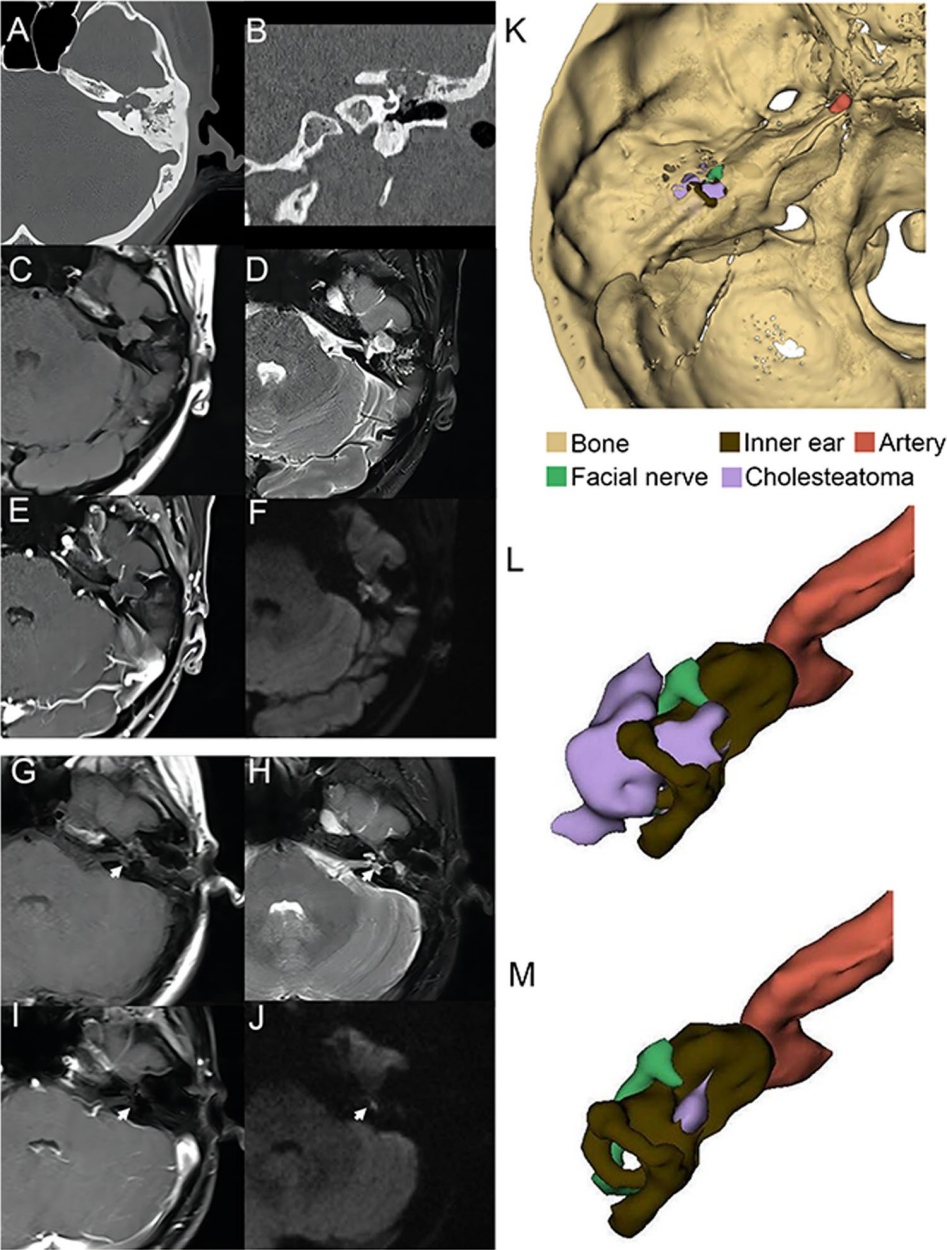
A case of cholesteatoma recurrence on imaging. A, B Left petrous bone lesion on preoperative CT involving the epitympanum, middle skull base, labyrinth, facial nerve, and internal auditory canal. C The mass had iso-intensity in T1W, D hyper-intensity in T2W, E annular enhancement, and F hyper-intensity in DWI. G–J A lesion (white arrow) appeared on MRI after the operation, characterized by iso-intensity in T1W, hyper-intensity in T2W, no enhancement, and hyper-intensity in DWI. K Preoperative three-dimensional reconstruction. L After hiding the bone, the lesion, inner ear, facial nerve, and internal carotid artery can be observed. M Postoperative three-dimensional reconstruction. *CT* computed tomography; *MRI* magnetic resonance imaging; *DWI* diffusion-weighted imaging; *T1W* T1-weighted; *T2W* T2-weighted
